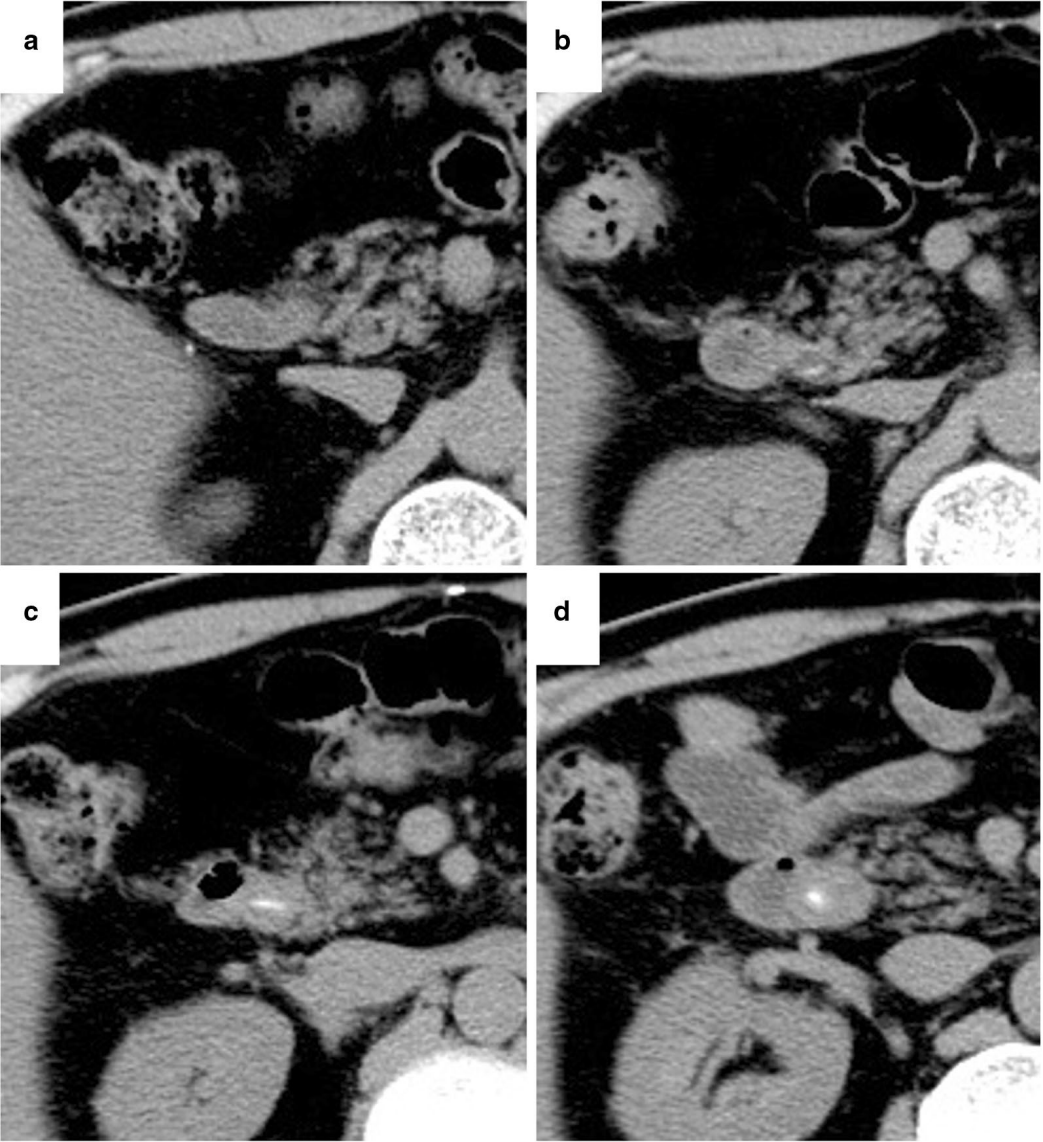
The time series of the surveillance computed tomography (CT) after gastrectomy. In 2012, 7 years after the patient underwent distal gastrectomy with Roux-en-Y reconstruction for gastric cancer, the common bile duct (CBD) stone was not detected (a). In 2015, at the past CT scan review, a spotted high-density area was recognized (b). The stone formation was also recognized in 2016, although the patient had no symptoms (c). CBD dilatation with stone formation causing obstructive jaundice was detected in 2017 (d)
